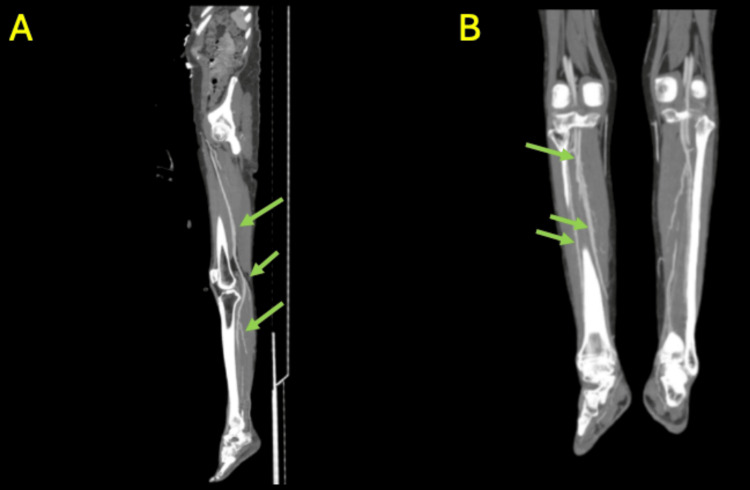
Computed tomography angiography of bilateral lower extremities In both sagittal view (A) and coronal view (B), there is preserved arterial flow bilaterally without large-vessel occlusion (denoted by green arrows).
